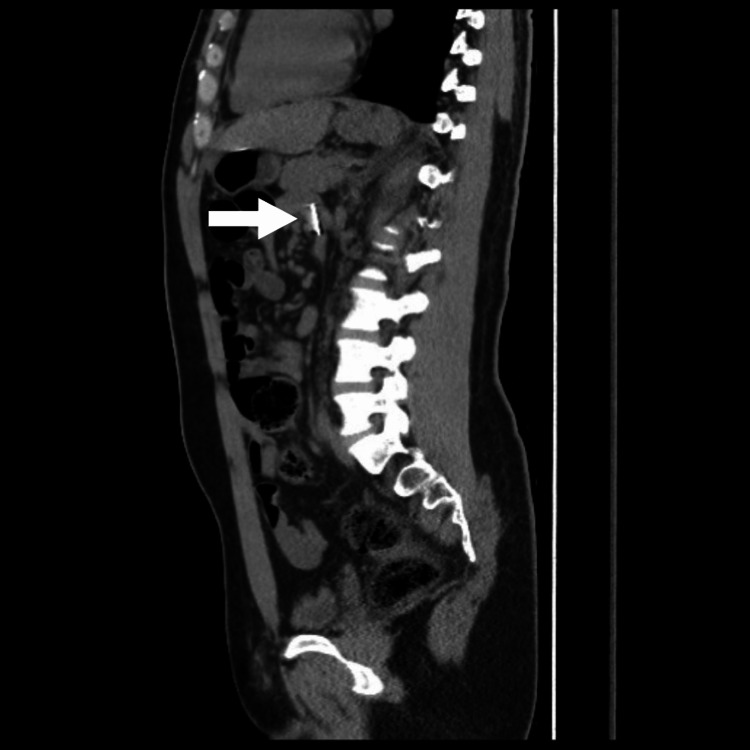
Computed tomography scan showing needle-shaped body in distal duodenum.
